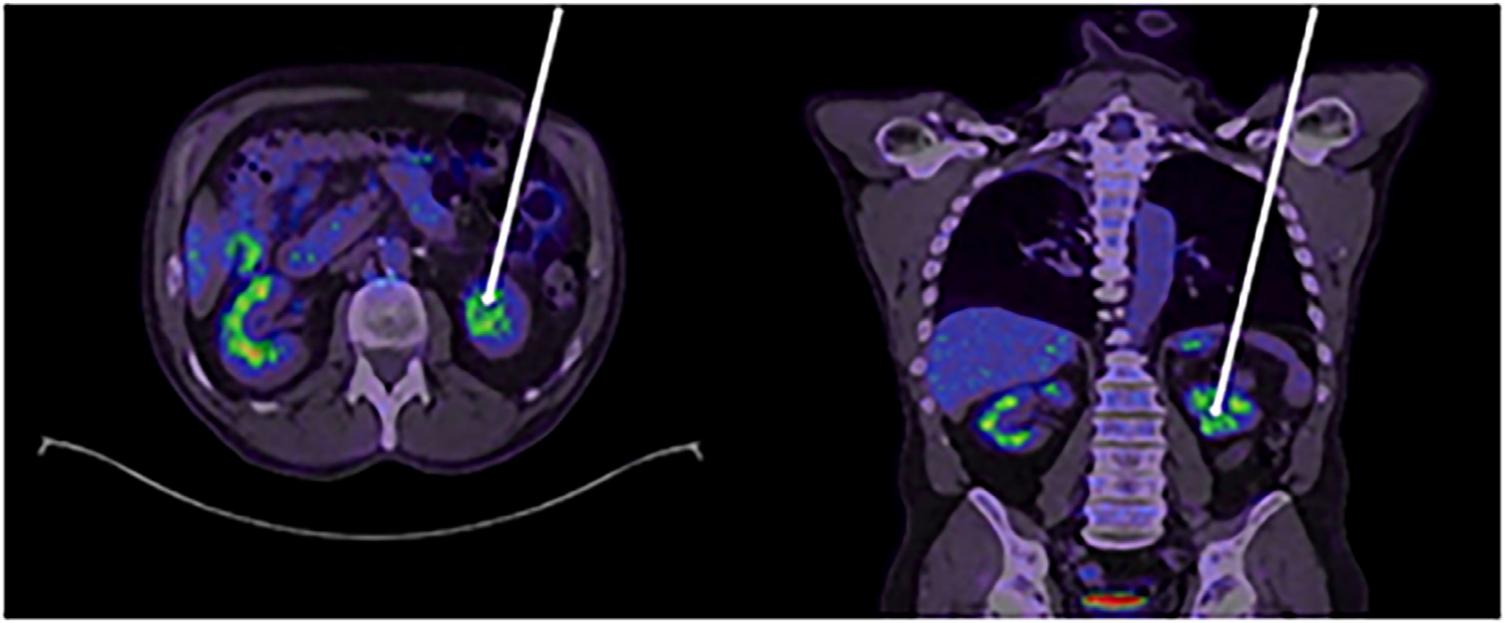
Panel 3a (axial an F-18 FDG PET CT) and Panel 3b (Coronal F-18 FDG PET CT) displaying high physiological uptake (as indicated by white arrows) in bilateral kidneys unable to clearly differentiate physiological uptake from Renal Cell Carcinoma or metastasis.
